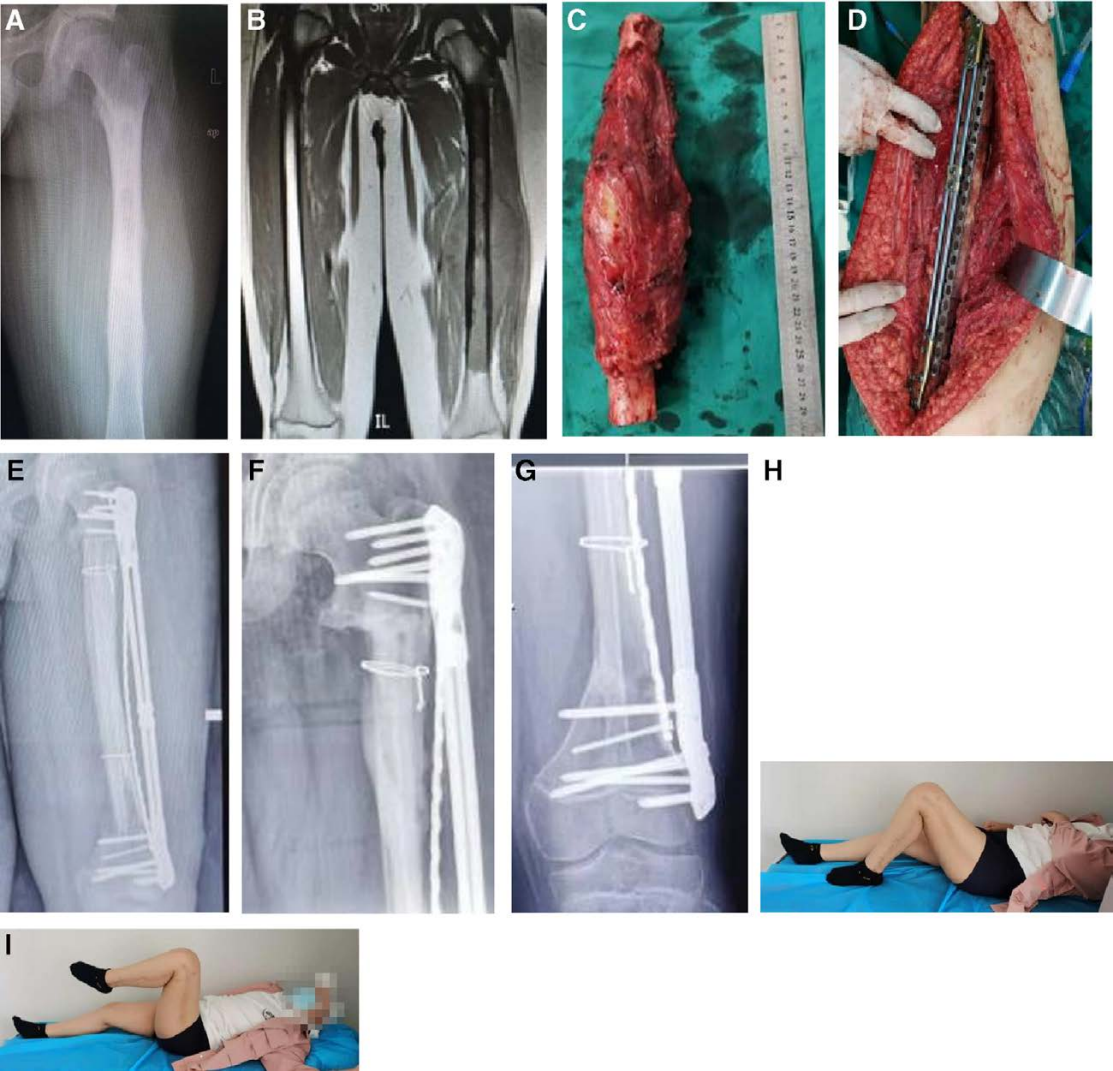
(A) The preoperative X-ray. (B) The preoperative MRI. (C) The focus of osteosarcoma in the middle femur was 30 cm long. (D) We anastomosed the vascularized fibula first, implanted the inactivated femur, and fixed it with 2 steel plates, finally. (E) Postoperative X-ray. (F and G) The X-ray showed 3 years after the operation. (H and I) The patient’s lower limb function was satisfactory. MRI = magnetic resonance imaging.
